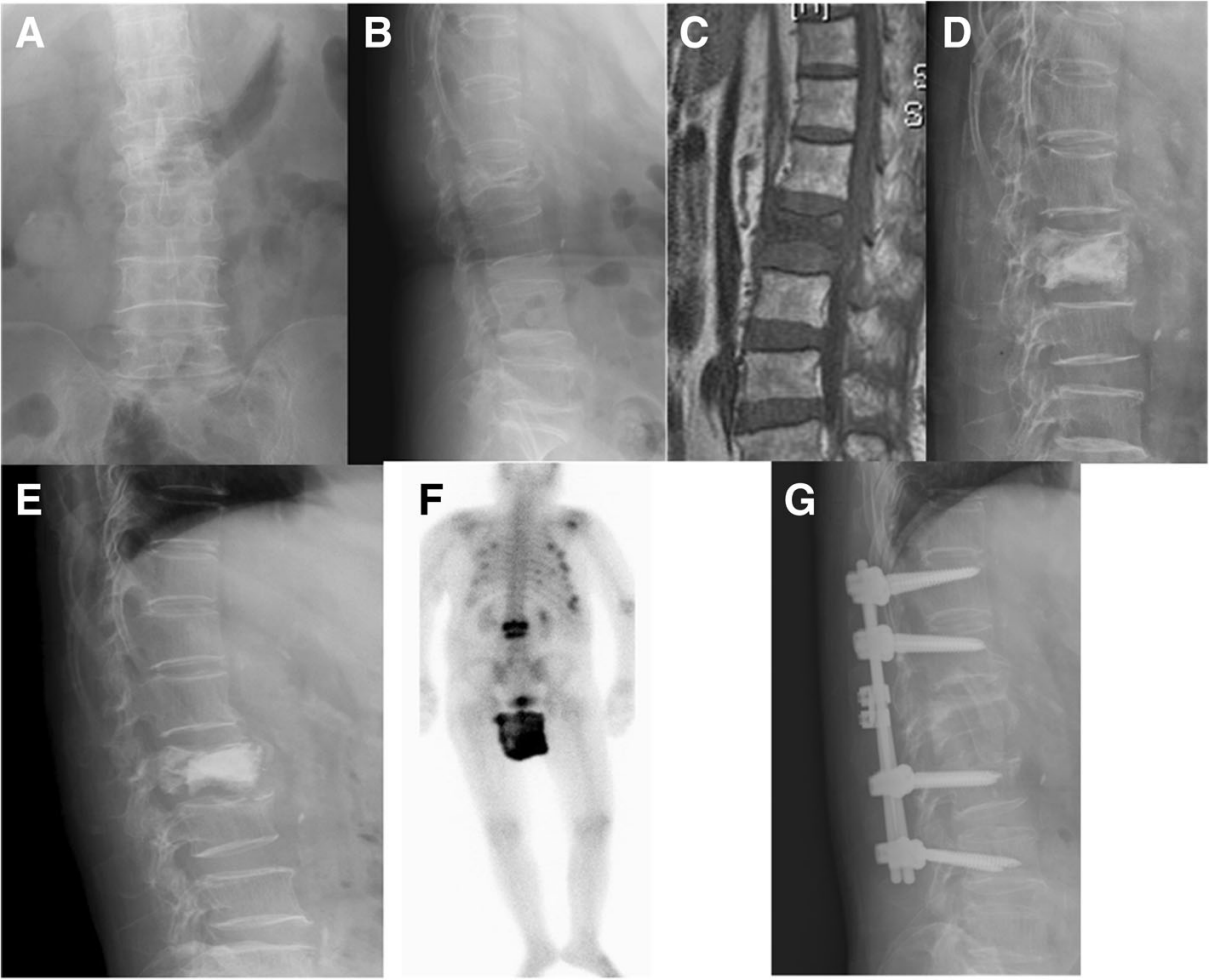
A case represented pyogenic spondylitis after VP. Initial radiographic images and MRI revealed L2 compression fracture (a, b, c). L2 VP was performed first (d). Svere low back pain was appeared again one month later. Plain radiograph showed L2 collapse with L2 lower endplate erosion (e). Gallium scan revealed L2–3 infectious spondylitis (f). Reconstruction revision surgery was performed (g). Tissue culture showed Coag (−) Staphylococcus
